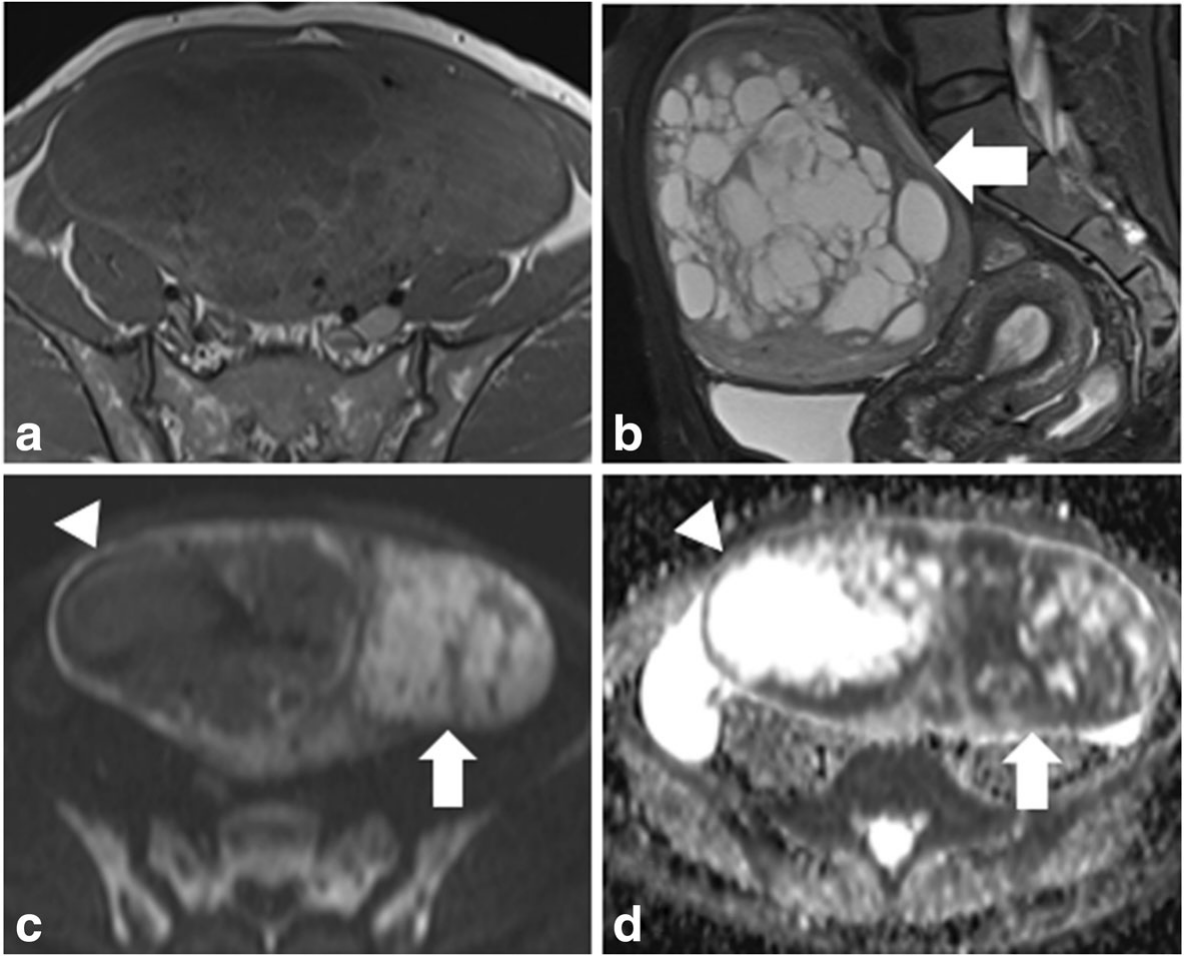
A 50-year-old woman with primary adult type OGCT (IIIa). (a) The spongelike changes (arrowhead) are observed in the interior of the tumor appearing as the mostly solid mass (b). The cystic contents (arrowhead) give the low signal on DWI (c) and relatively high signal on ADC map (d), while the solid part (arrow) with the relatively high signal on DWI and low signal on ADC map
